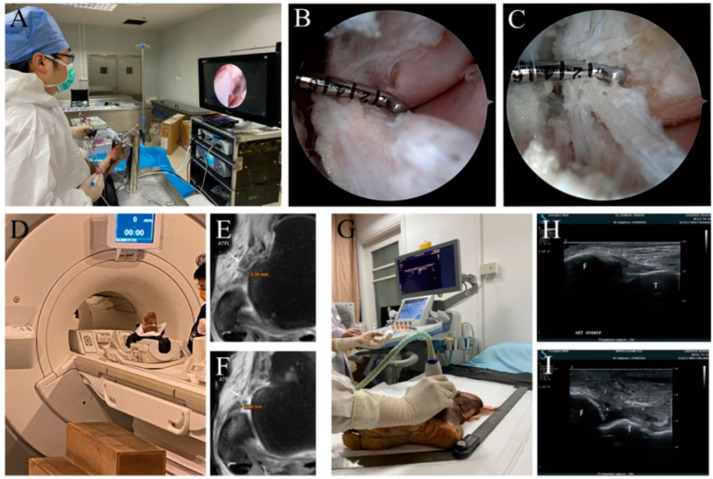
Establishment of most ATFL injury models. (A–C): the severe ATFL injury model was established under total arthroscopy. (D–F): MRI confirmed successful modeling before and after operation. (G–I): B-ultrasound confirmed successful modeling before and after operation. MRI: magnetic resonance imaging.
